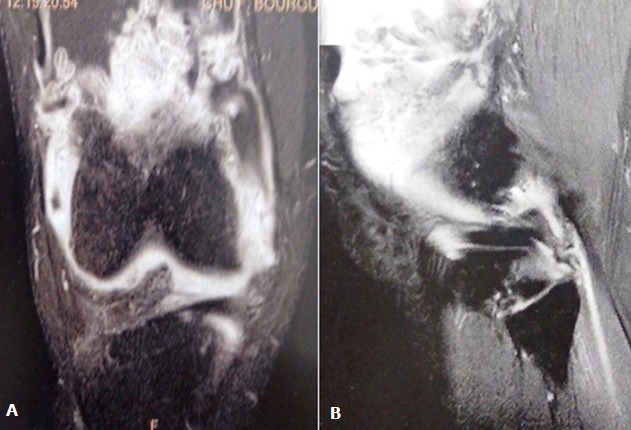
Magnetic resonance imaging: the frontal (A) and sagittal (B) sections of the right knee showing hyper-signal synovial proliferation which predominates at the quadriceptal recess
